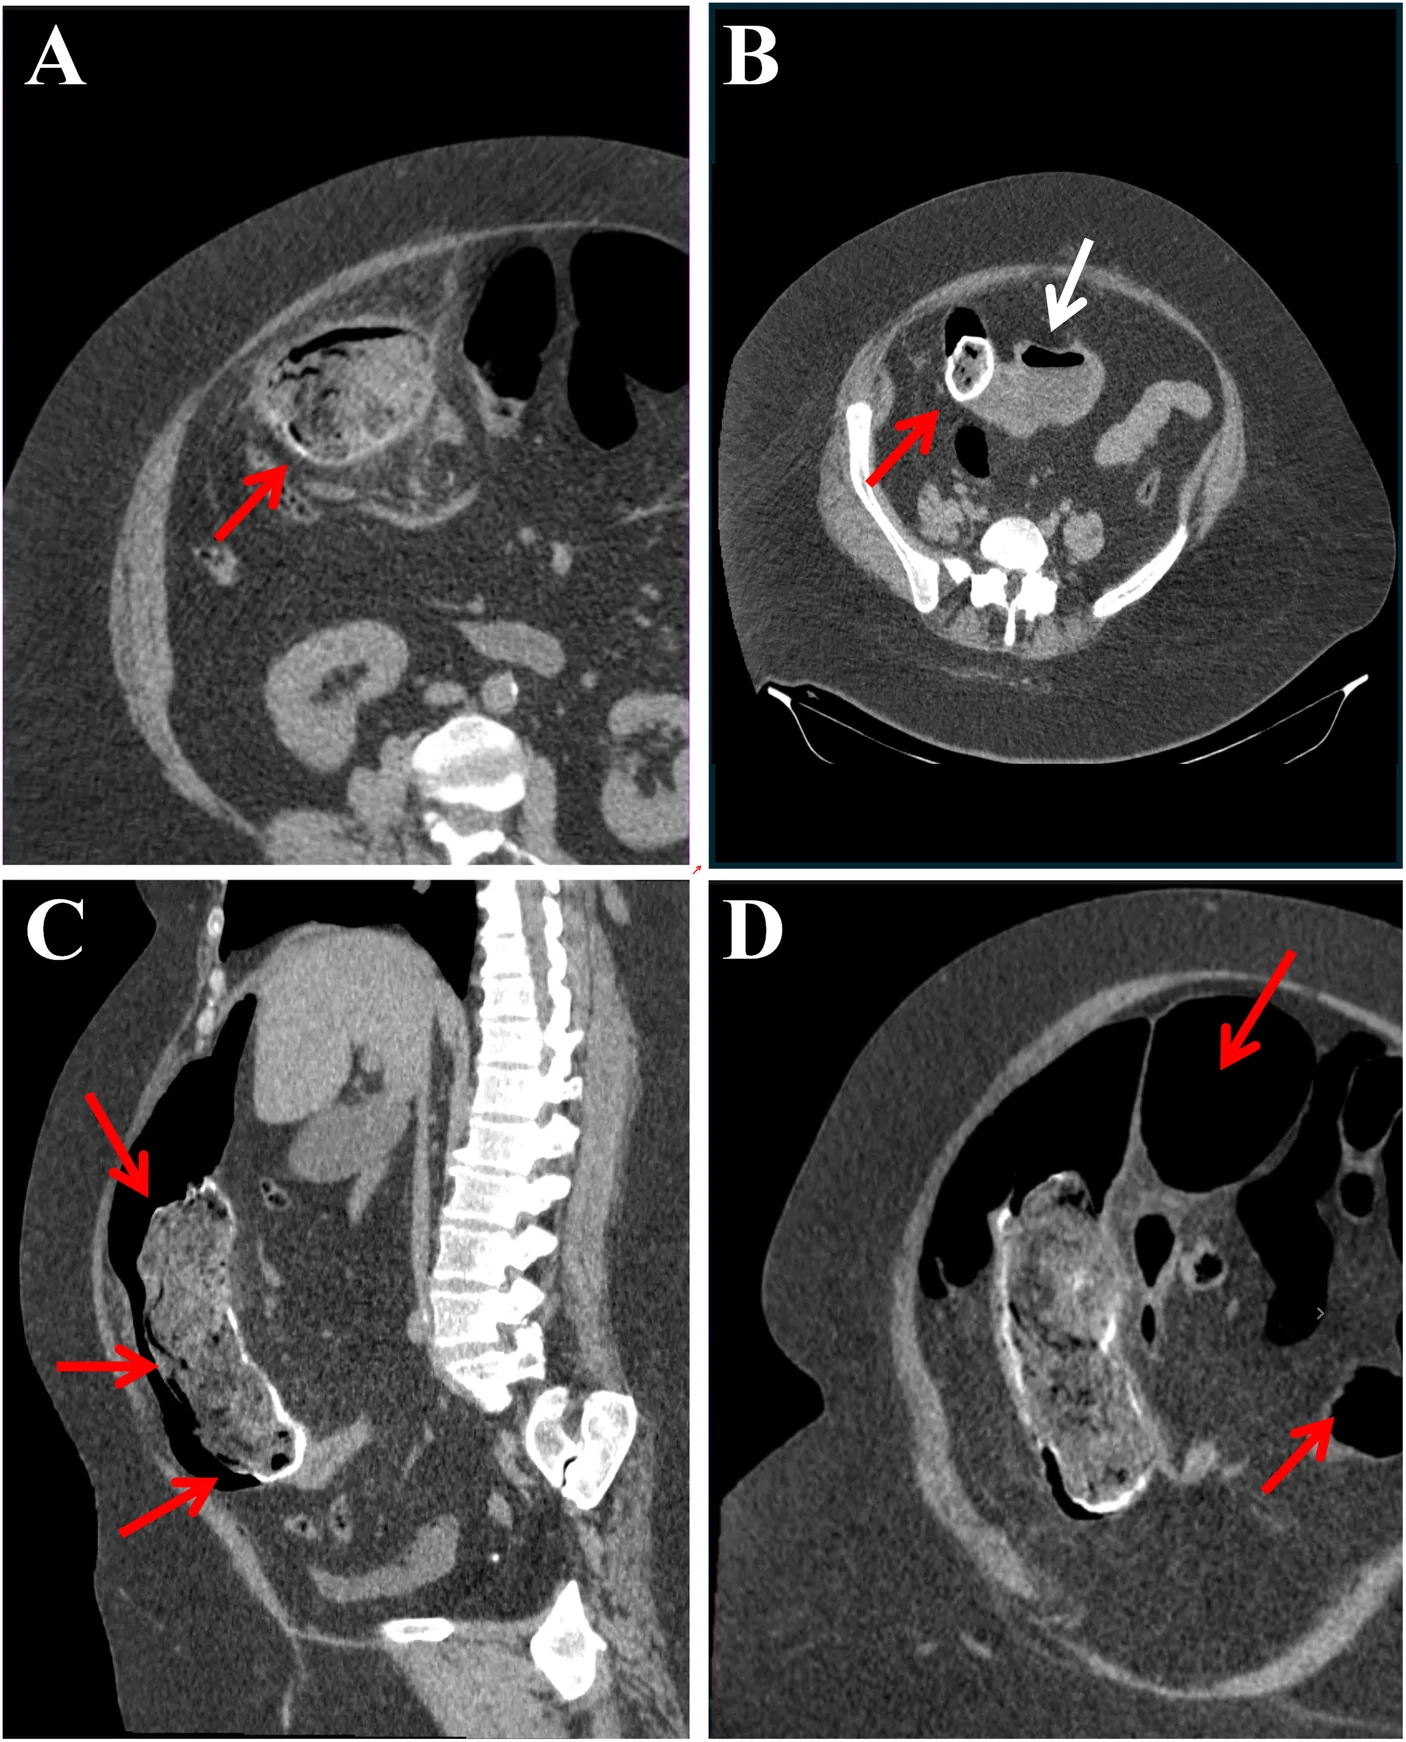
Unenhanced multiplanar abdominopelvic CT at admission. The study was acquired in the unenhanced phase: intravenous iodinated contrast was withheld because of the acute kidney injury present on admission in a hypotensive, hypovolaemic patient, and enteral contrast was not administered because of persistent vomiting and clinical obstruction. (A) Axial image at the level of the lesion showing the intraluminal mass with a mottled, spongiform internal pattern containing entrapped gas (red arrow); the wall of the affected ileal loop is thickened, and the adjacent mesenteric fat shows stranding with laminar free fluid, indicating compromise of the bowel wall. (B) Axial image acquired caudal to the mass, demonstrating the obstructive intraluminal lesion at the transition point (red arrow), with proximal small-bowel dilatation and an air–fluid level (white arrow). (C) Oblique sagittal reformat showing the craniocaudal extent of the intraluminal textiloma in the right flank (red arrows), with hyperdense material along its postero-inferior margin and edema of the adjacent mesenteric fat. (D) Oblique coronal reformat demonstrating retrograde dilatation of the proximal small bowel with abundant intraluminal gas (red arrows). No radio-opaque marker attributable to a surgical textile was identified on any plane. Because the examination was unenhanced, capsular enhancement and mural perfusion could not be evaluated.
